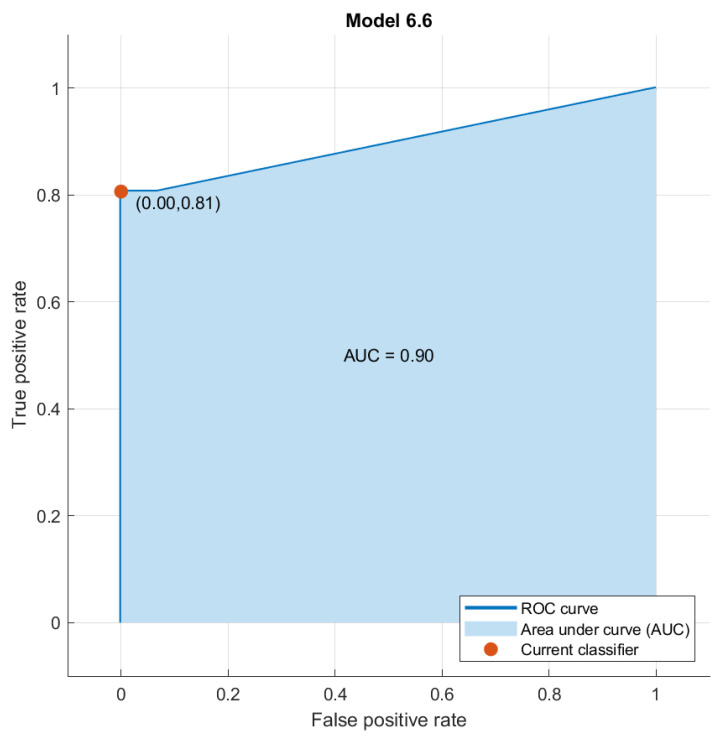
AUC of the best classifier (KNN) trained with all significant radiomic features extracted from each MRI sequence.
